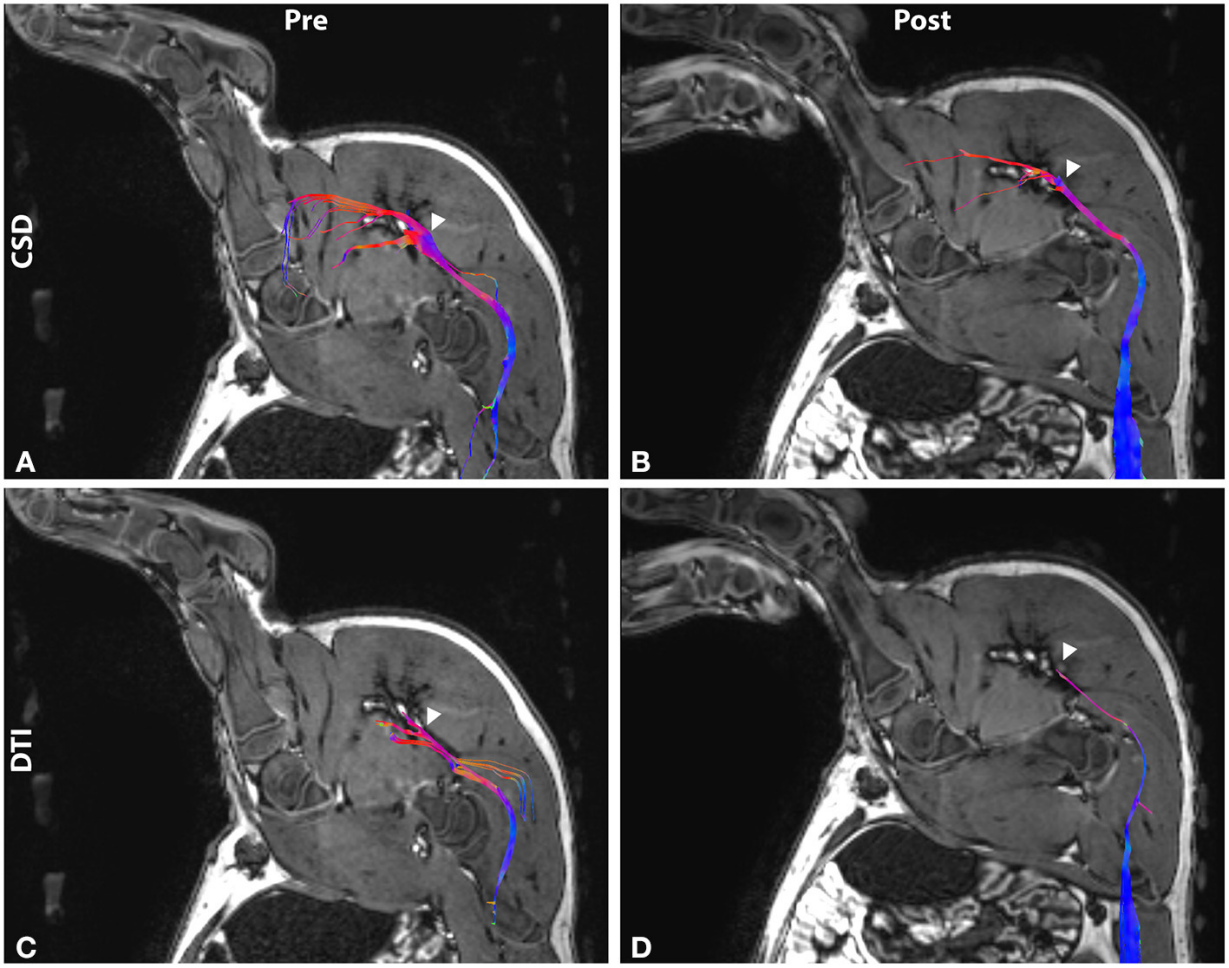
Tractography before and after MRgFUS treatment in sagittal view. Tracts are reconstructed using (A,B) constrained spherical deconvolution (CSD) and (C,D) diffusion tensor imaging (DTI) methods. Tracts are overlaid on T1-weighted images and colored by orientation: anterior-posterior (blue); superior-inferior (red); left-right (green). Arrowhead indicates treatment target.
